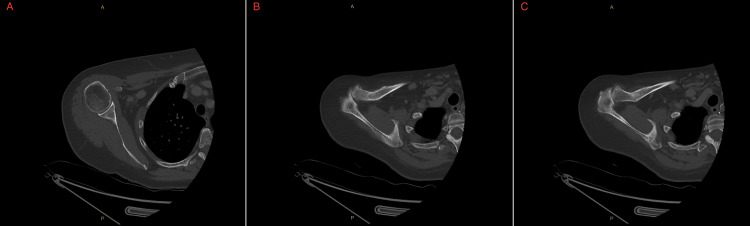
Axial CT Bone Window of the Humerus With IV Contrast Axial CT images of the right humerus in bone window (with IV contrast), demonstrating key osseous findings. (A) Axial view through the glenohumeral joint shows mild-to-moderate joint space narrowing and subchondral sclerosis, consistent with glenohumeral osteoarthritis. No fracture, dislocation, or effusion is seen. (B, C) Axial views through the acromioclavicular joint demonstrate moderate-to-severe joint space narrowing, osteophyte formation, and cortical irregularity, consistent with advanced acromioclavicular osteoarthritis. No osseous lesions or periarticular collections are present.
